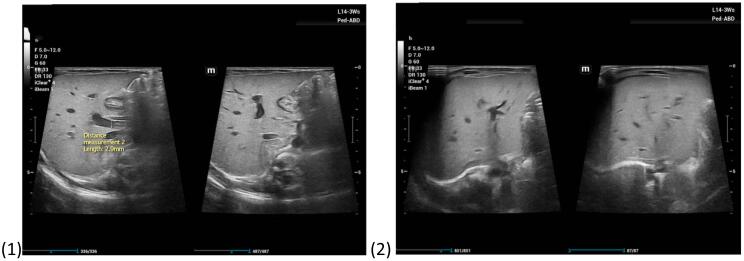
Ultrasound images on admission.
